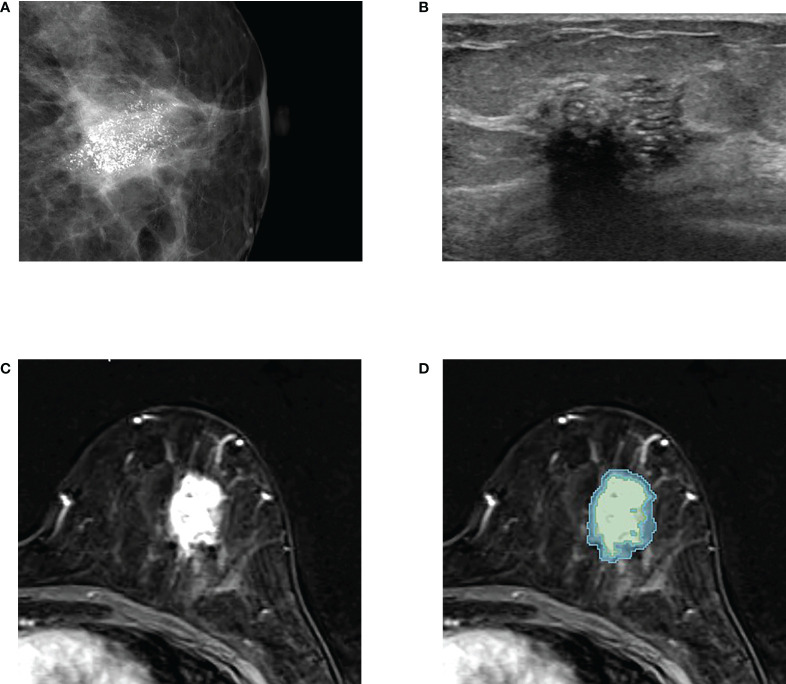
An example of tumor segmentation in a 50-year-old woman who complained of palpable lump in her left breast, which was pathologically confirmed as upgraded ductal carcinoma *in situ* (DCIS) after surgical excision. (A) Mammography shows about 3 cm extent of fine pleomorphic microcalcifications seen in the left central breast. (B) Ultrasound image shows the non-mass lesion with microcalcifications. (C) Axial first postcontrast T1-weighted image with subtraction shows a 3 cm irregular heterogeneously enhancing mass in left lower central breast. (D) The green and blue shadings represent the intratumor and peritumor ROIs, respectively, which are created by 3D slicer.
